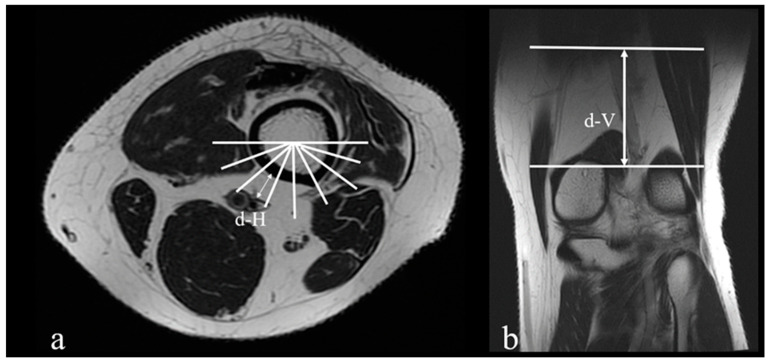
The example of the measurements in magnetic resonance images of a 56-year-old female: (a) the closest distance (d-H) between the popliteal vessels to the femoral cortex is 14.65 mm, and the position of the popliteal vessels adjacent to femoral cortex is section C; (b) the distance (d-V) between the AT and the axial level of “d-H” is 41.08 mm.
